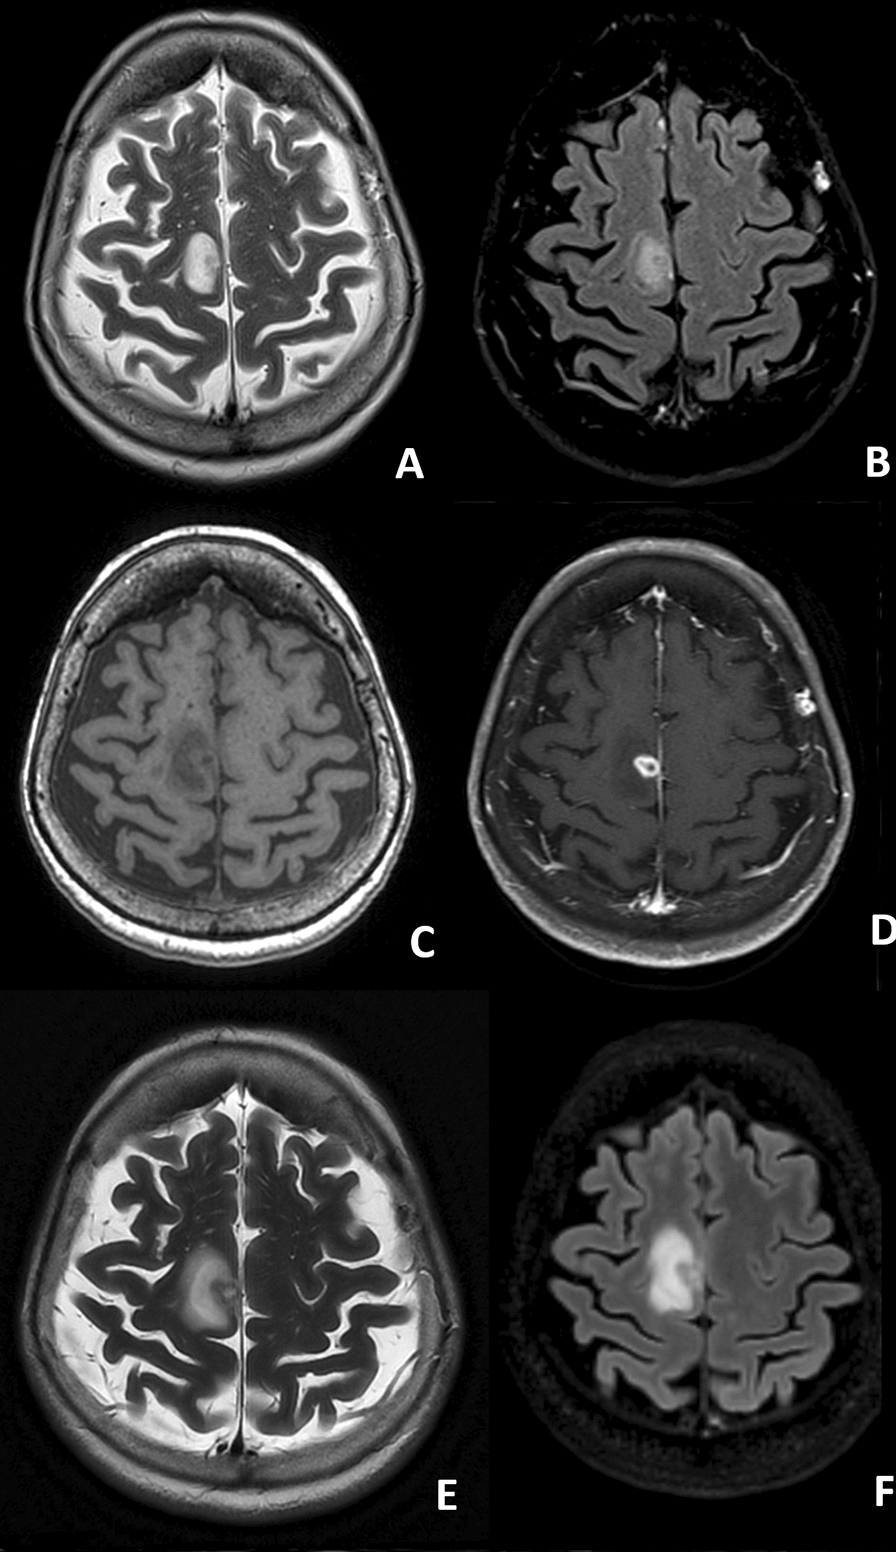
Diagnostic imaging in MRI: A axial T2‑weighted turbo spin echo (TSE) and B axial fluid-attenuated inversion recovery (FLAIR) images showing a hyperintense lesion with well‑defined margins and no evidence of perilesional edema. C–E Images acquired after steroid therapy: C axial T1-weighted image showing a mild increase in the size of the expansile lesion, with more indistinct margins; D post-contrast image demonstrating the appearance of a new nodular component within the lesion, exhibiting contrast enhancement; E axial T2‑weighted and B axial FLAIR images documenting the appearance of subtle perilesional edema
